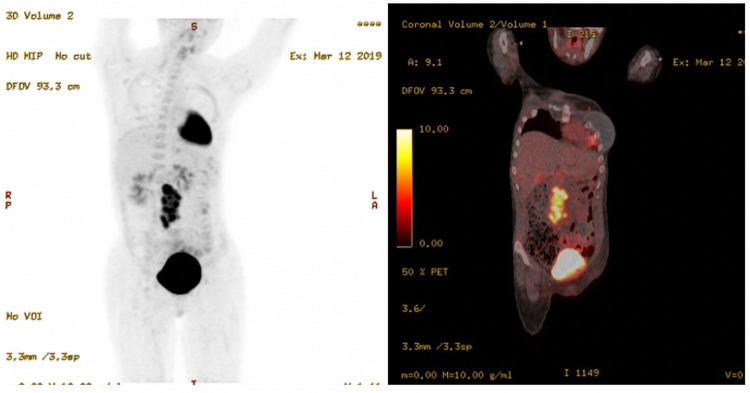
PET-CT showing interval increase in the extent and metabolic activity of the central abdominal lobulated nodal conglomerate mass, and a new hypermetabolic retroperitoneal lymph node that was confirmed by biopsy to be a metastatic immature ovarian teratoma. PET-CT: positron emission tomography-computed tomography
